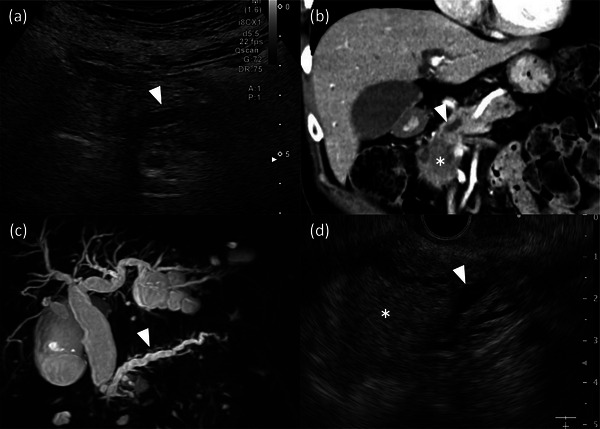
Image findings of the main pancreatic duct dilation (MPD Dil.) (a) abdominal ultrasonography findings MPD Dil. (arrowhead, MPD Dil.). (b) CT scan showing MPD Dil. because of stenosis with pancreatic head cancer: Arrowhead, MPD Dil.; asterisk, cancer. (c) Magnetic resonance cholangiopancreatography findings showing MPD Dil.: Arrowhead, MPD Dil. (d) EUS findings showing MPD Dil. caudal to cancer: arrowhead, MPD Dil.; asterisk, cancer. Cases with any of these findings were considered to be MPD Dil.
